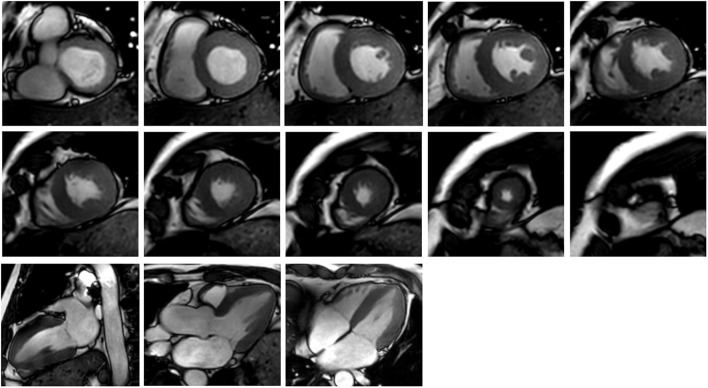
Cardiac MR images corresponding to 10 short-axis slices (the upper two rows) and three long-axis slices (the third row).
